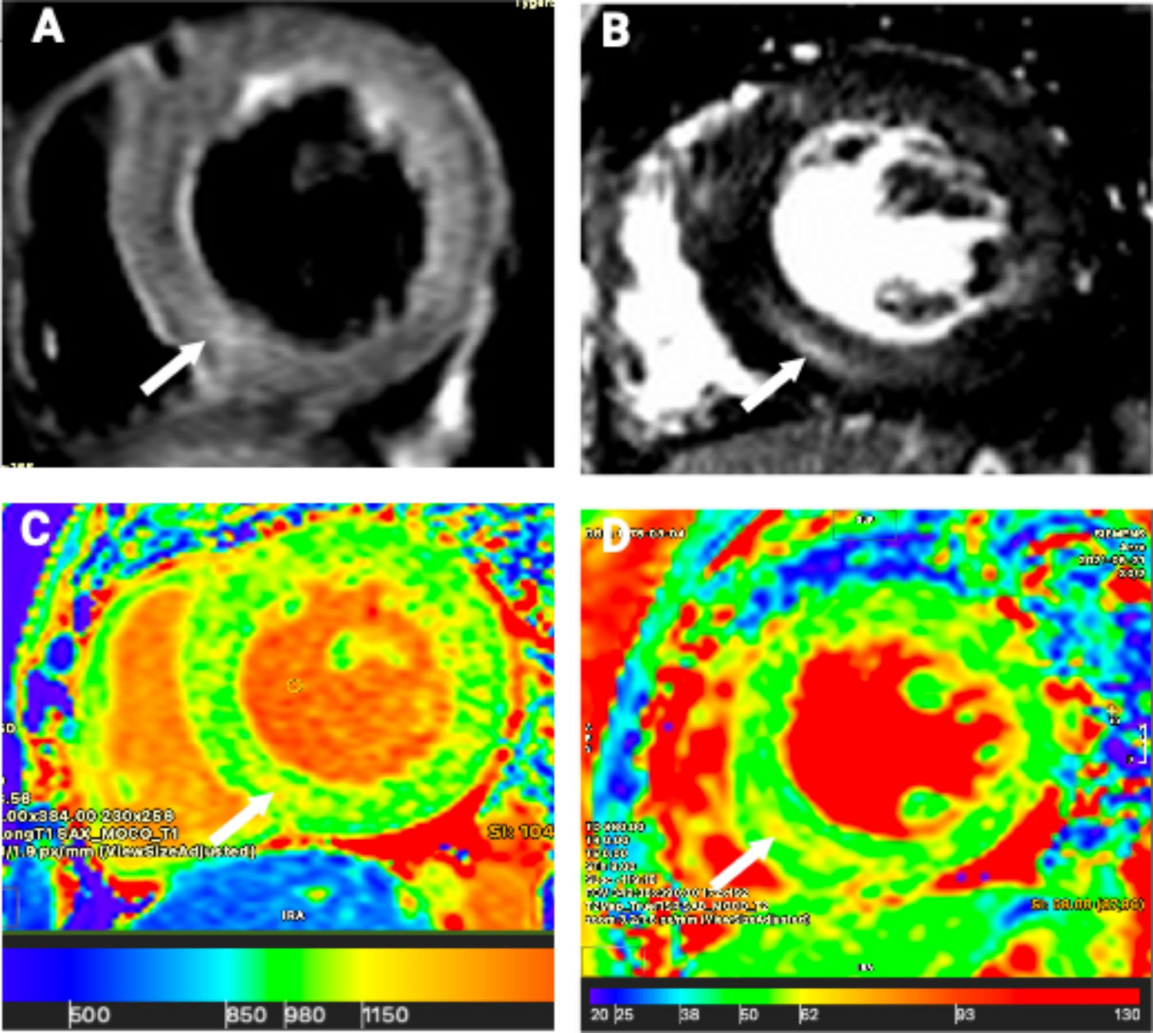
Exemplar images of myocardial oedema demosntrated using various sequences. White arrows demonstrate areas of abnormality. (A) Increased T2-weighted signal intensity; (B) non-ischaemic late gadolinium enhancement; (C) increased native T1; (D) increased T2^29^.
